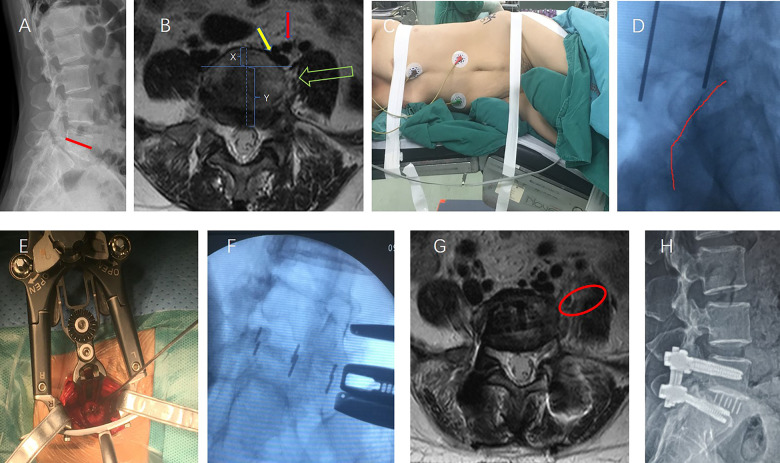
A 47-year-old woman underwent XLIF at the L5/S1 level. (A) Preoperative radiograph showed L5 degenerative spondylolytic spondylolisthesis, and the iliac crest was below the 1/2 line of the L5 vertebra (the red line). (B) The picture shows that the XLIF approach at L5-S1 in this case (green arrow) was feasible without obstruction of the left common iliac vein (yellow arrow) and the left iliac artery (red arrow). The iliac vessels were located in the first quarter of the intervertebral space (X:Y < 1:3). (C) Surgical position. (D) The iliac crest (red line) is lowered below the L5/S1 intervertebral space. (E) Intraoperative photograph showing the L5–S1 disk was exposed. (F) Intraoperative fluoroscopy after the implantation of the cage. (G) The red circle shows the working channel through the left psoas at L5/S1. (H) Radiographs taken 3 months postoperatively showed slip reduction with good cage positioning.
